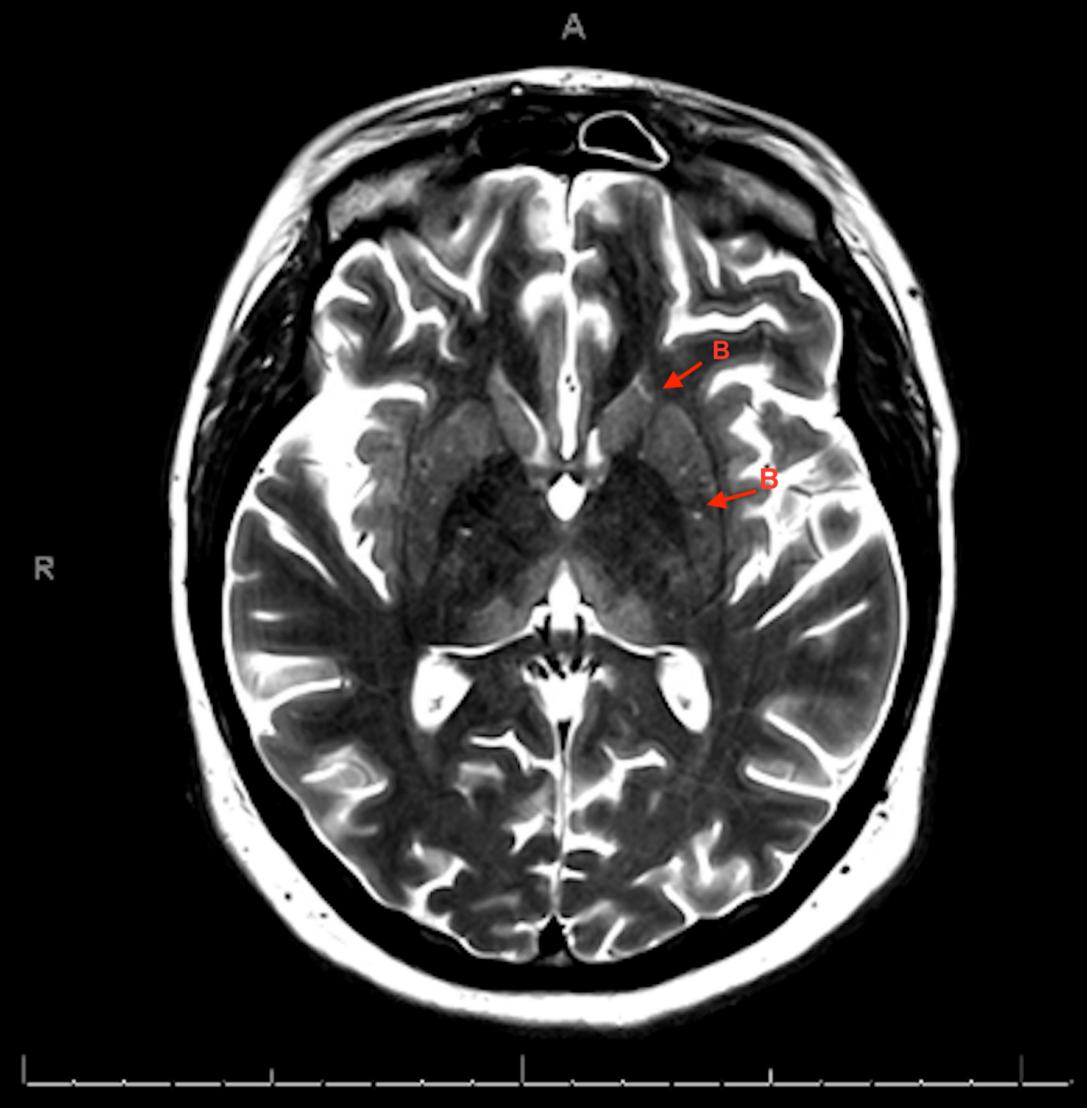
MRI of the brain. T2 sequence showing symmetric bilateral hyperintensities in the basal ganglia structures (B) marked with red arrows
